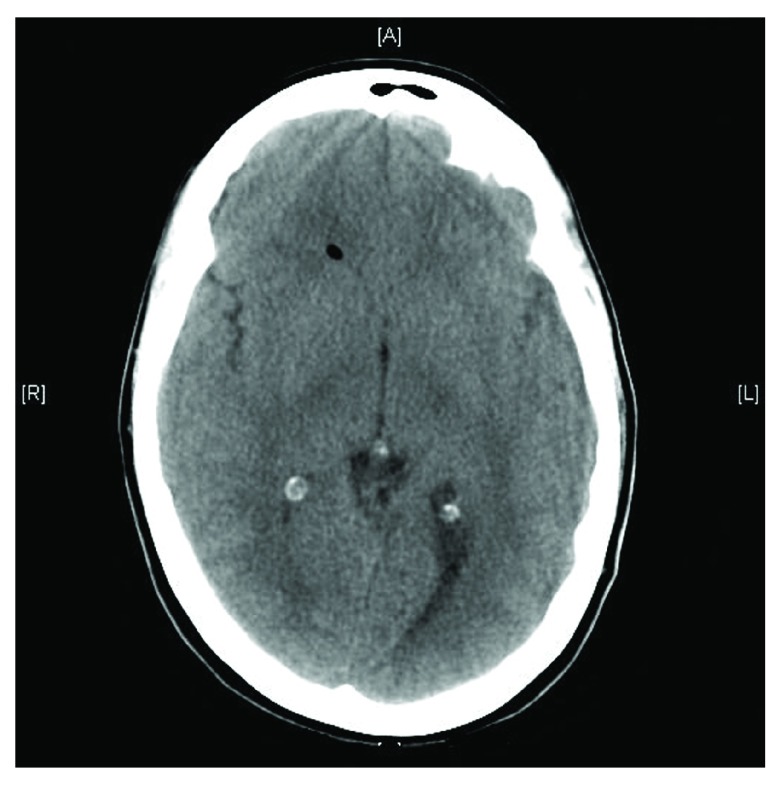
Axial CT scan shows the presence of a gas bubble in the ventricular system following dural puncture, in the right frontal cistern horn of the lateral ventricle.
